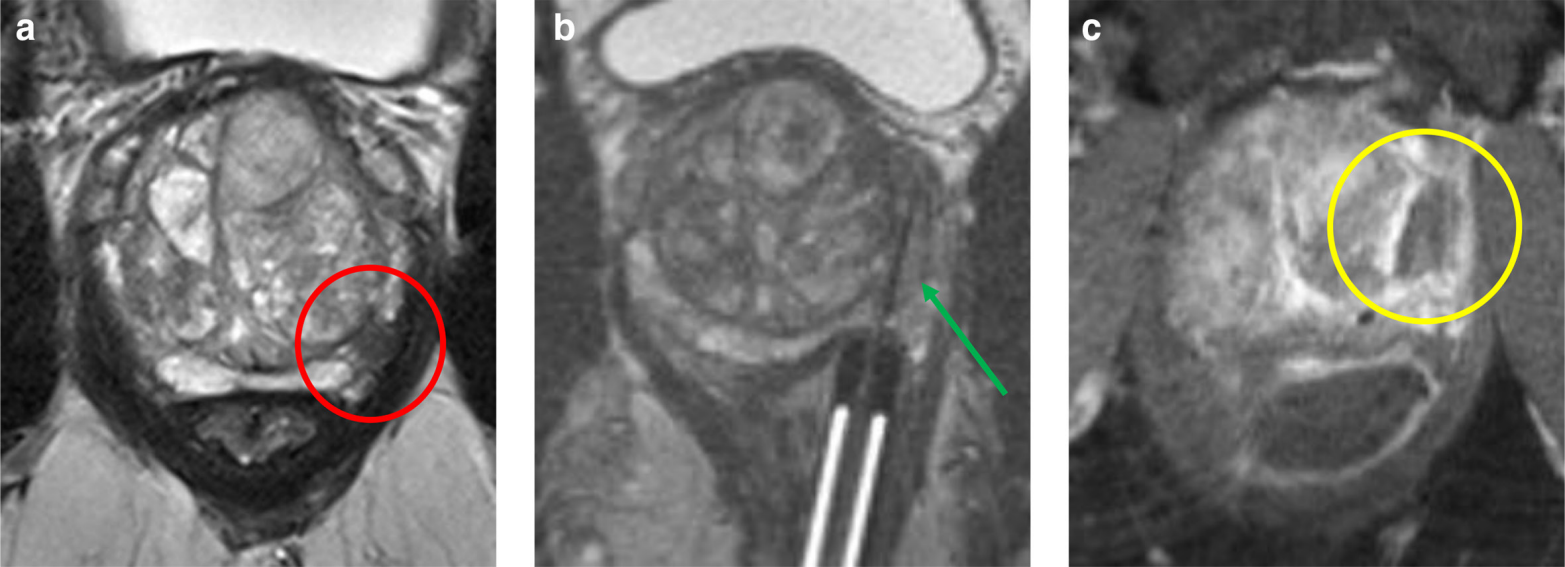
Transrectal focal laser ablation MRI of a 69-year-old male with an initial PSA of 14.5 ng ml^−1^ that underwent transrectal FLA as treatment for a *de novo* lesion (Gleason score 3+4=7) at the left peripheral zone (PI-RADS 4). (a) Axial *T*
